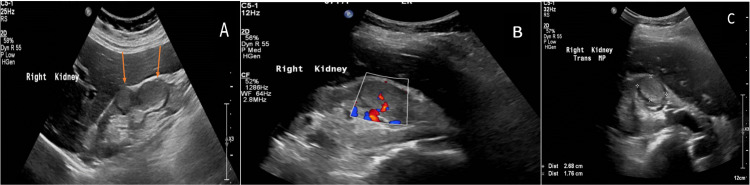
A, B, and C: Sagittal and transverse greyscale and Doppler ultrasound images of the right kidney showing two lobulated hyperechoic masses of the interlobar region and lower pole (arrows).
